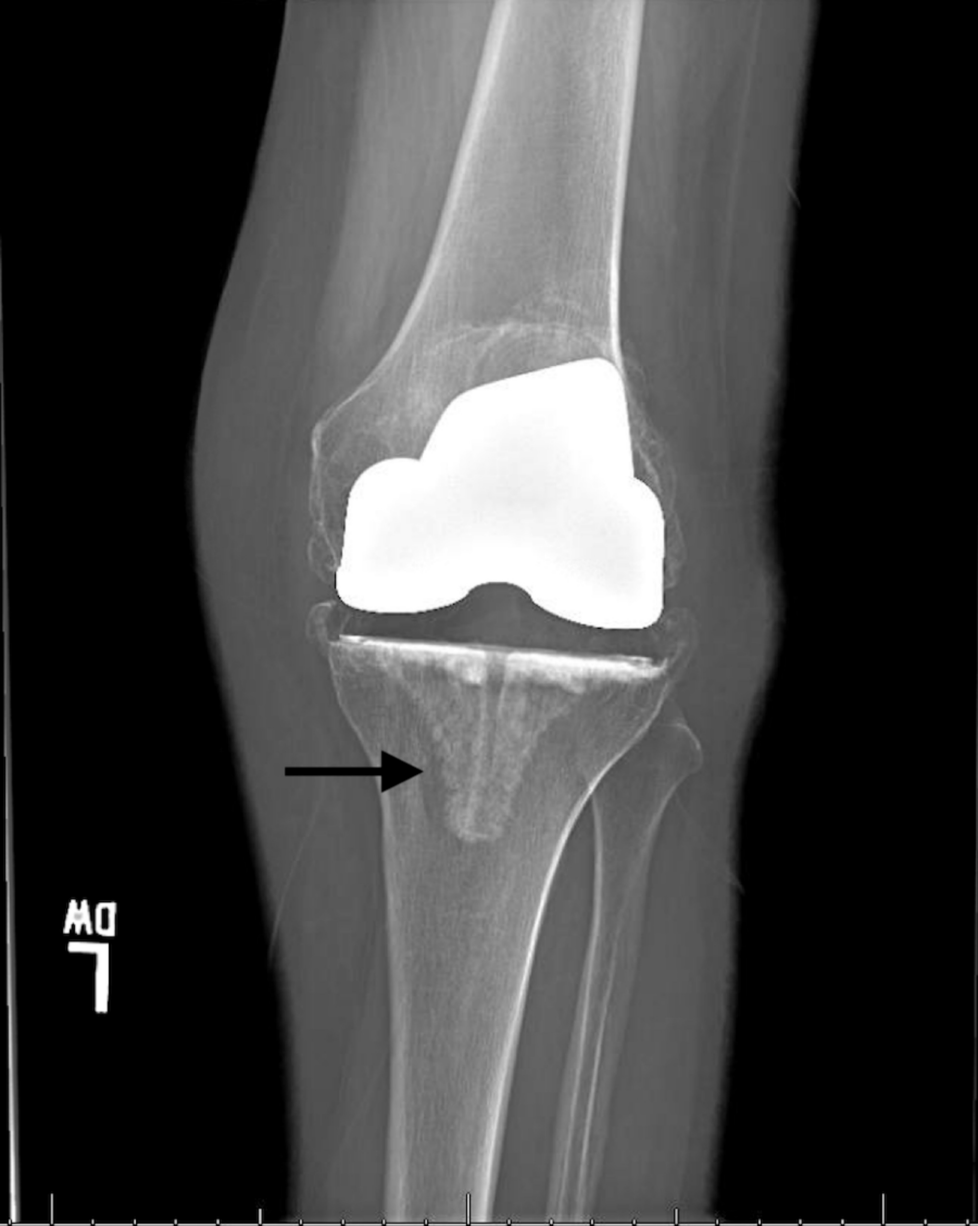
Anteroposterior radiograph of left knee at presentation showing lysis and subsidence of tibial component
